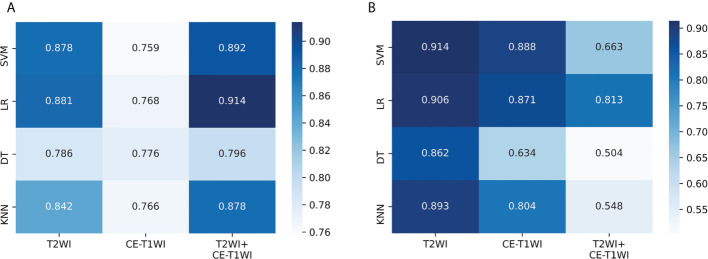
Performance of twelve machine learning models on the two test sets. (A) The AUC values in the test set 1. (B) The AUC values in the test set 2. SVM, support vector machine; LR, logistic regression; DT, decision tree; KNN, k-nearest neighbor; T2WI, T2-weighted image; CE-T1WI, contrast–enhanced T1-weighted image; T2WI+CE-T1WI, the combination of T2-weighted image and contrast–enhanced T1-weighted image.
